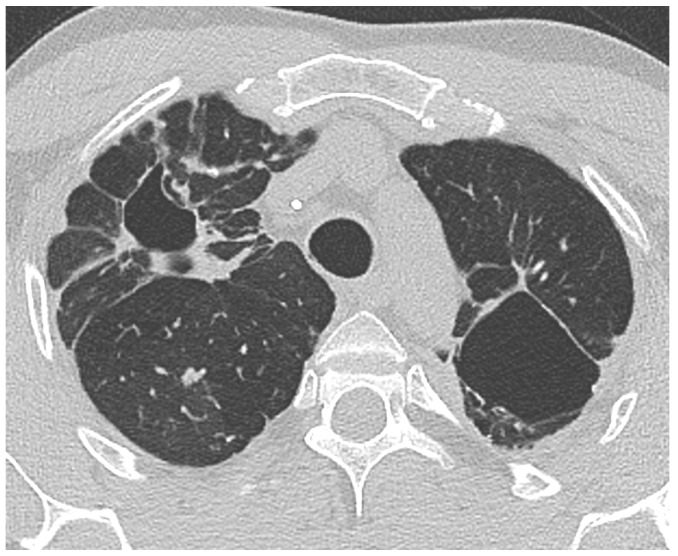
Chest CT: multiple thin-walled cavitary lesions in both upper lobes, consistent with active pulmonary tuberculosis. Representative image from the anonymized institutional radiology archive of University Hospital Frankfurt.
